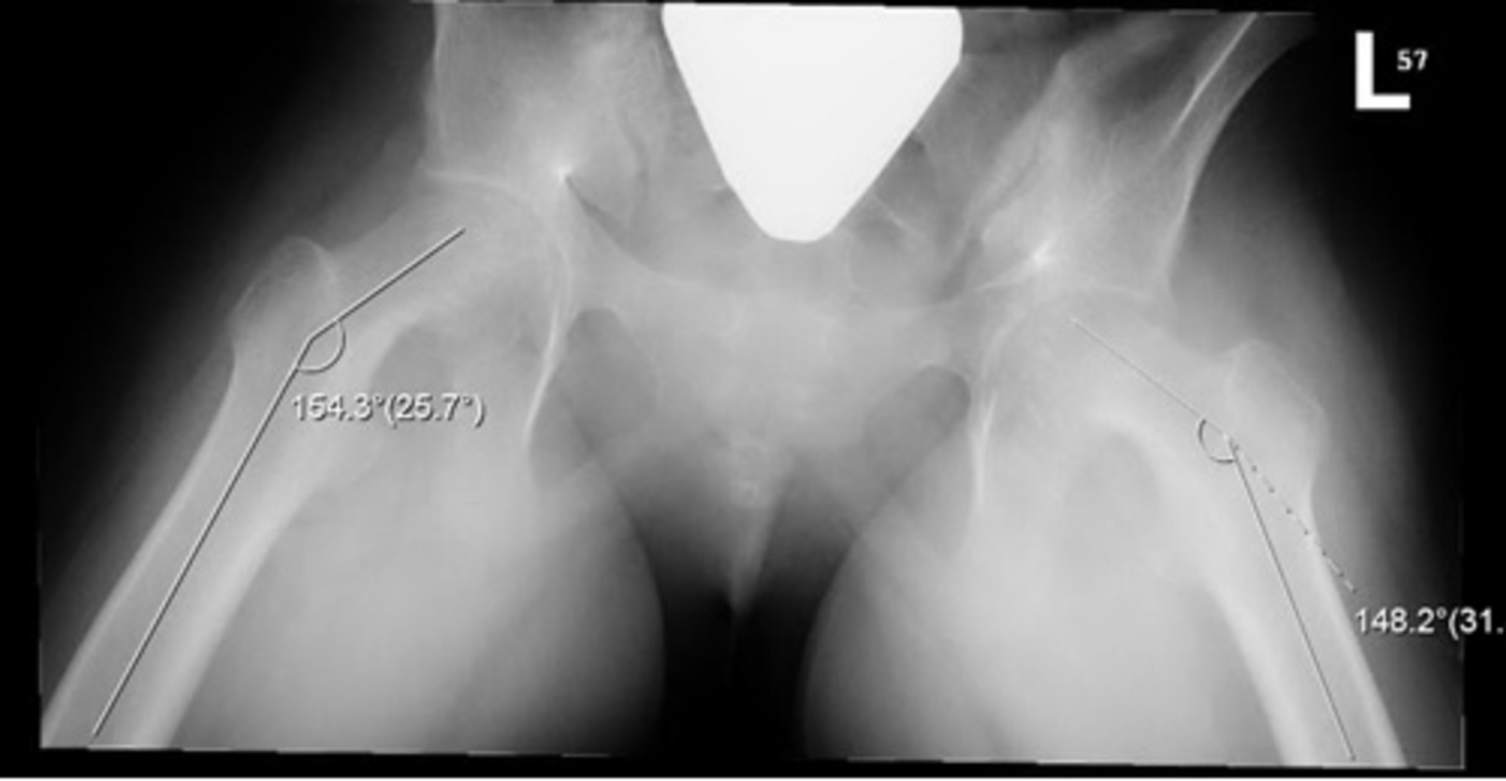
Frog-leg lateral radiograph The radiograph showed bilateral SCFE with valgus deformity. The right and left femoral neck-shaft angles measured 154.3 and 148.2 degrees, respectively. SCFE: slipped capital femoral epiphysis
